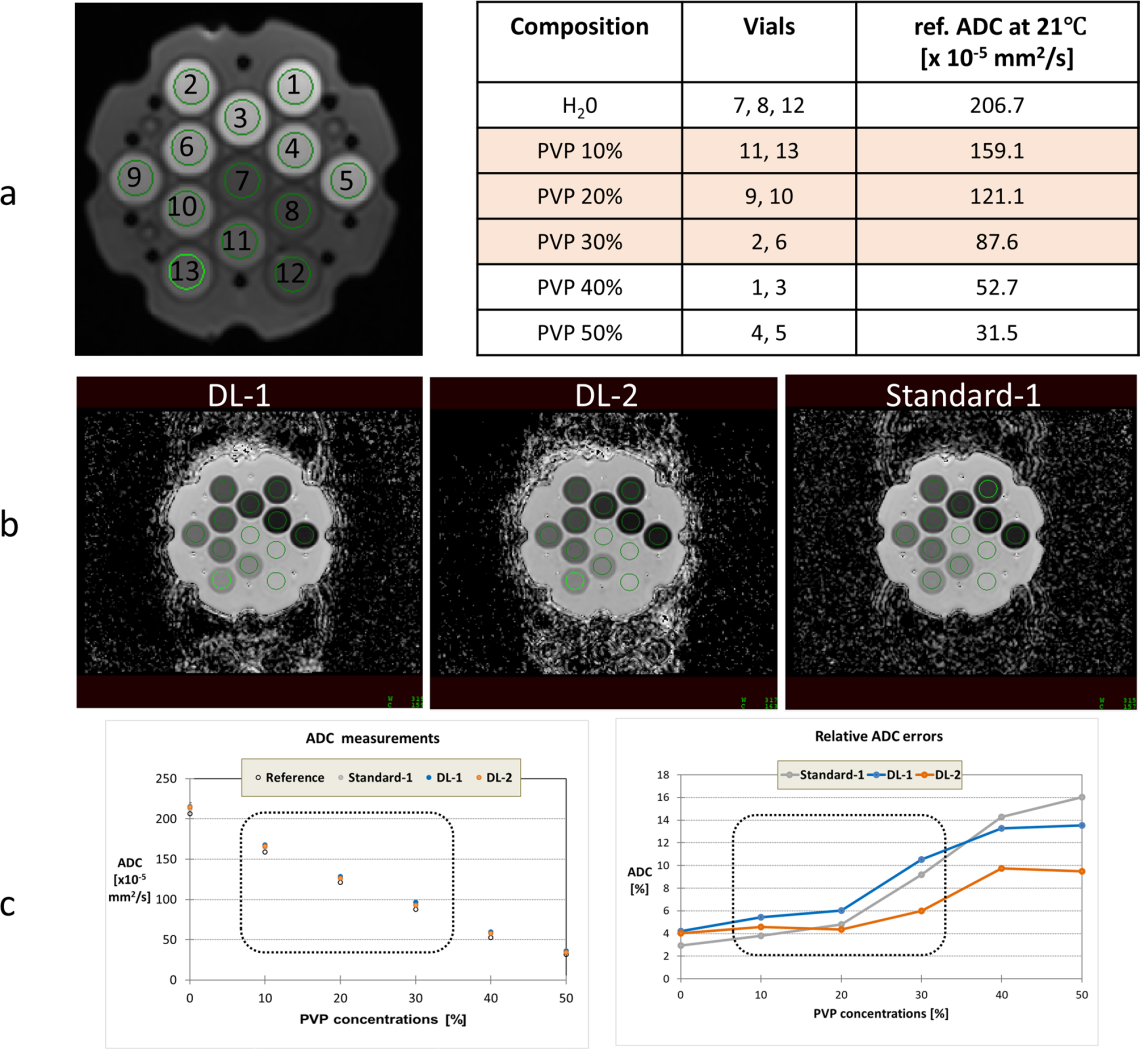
Test-object structure and vials composition (a), ADC maps derived from the three DWI series (b), and corresponding measured ADC values and their percentual relative error (c). Range of ADC values of biological interest is highlighted in cream (panel a) and by a rectangle in panel c. PVP = polyvinylpyrrolidone; ADC = apparent diffusion coefficient; DL-1 = moderately-accelerated DWI using a deep-learning reconstruction algorithm; DL-2 = highly-accelerated DWI using a deep-learning reconstruction algorithm; Standard-1 = moderately-accelerated DWI using a standard reconstruction algorithm
